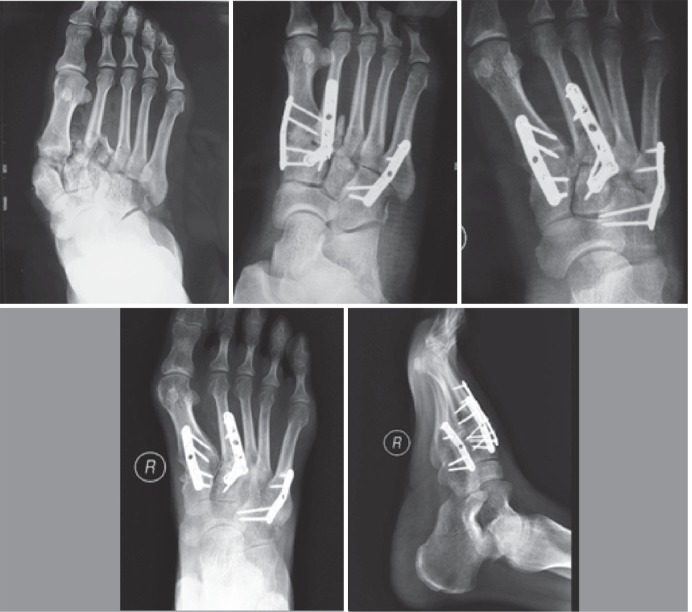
Case 1: Female 38 yer as old Myerson I type, X-radiograph before operation; X-radiograph shows the fracture-dislocation healed two weeks and six months post-operation.
